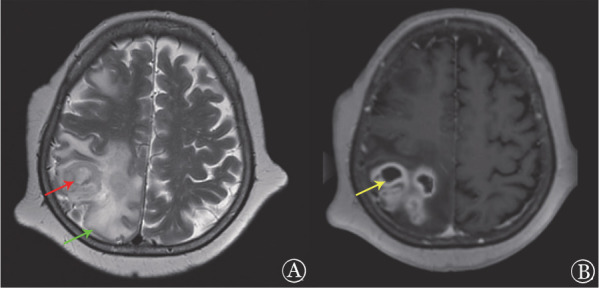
慢性淋巴细胞白血病患者（例1）发生中枢神经系统侵袭性真菌病的头颅增强核磁共振（MRI）表现 A MRI轴位T2，右侧额顶叶皮层及皮层下见多发结节状长短混杂T2信号，增强扫描呈变化强化（红色箭头），病灶周围见指状水肿包绕（绿色箭头）；B MRI轴位T1，可见囊腔，囊壁厚薄均匀，未见明确壁结节样强化（黄色箭头）
